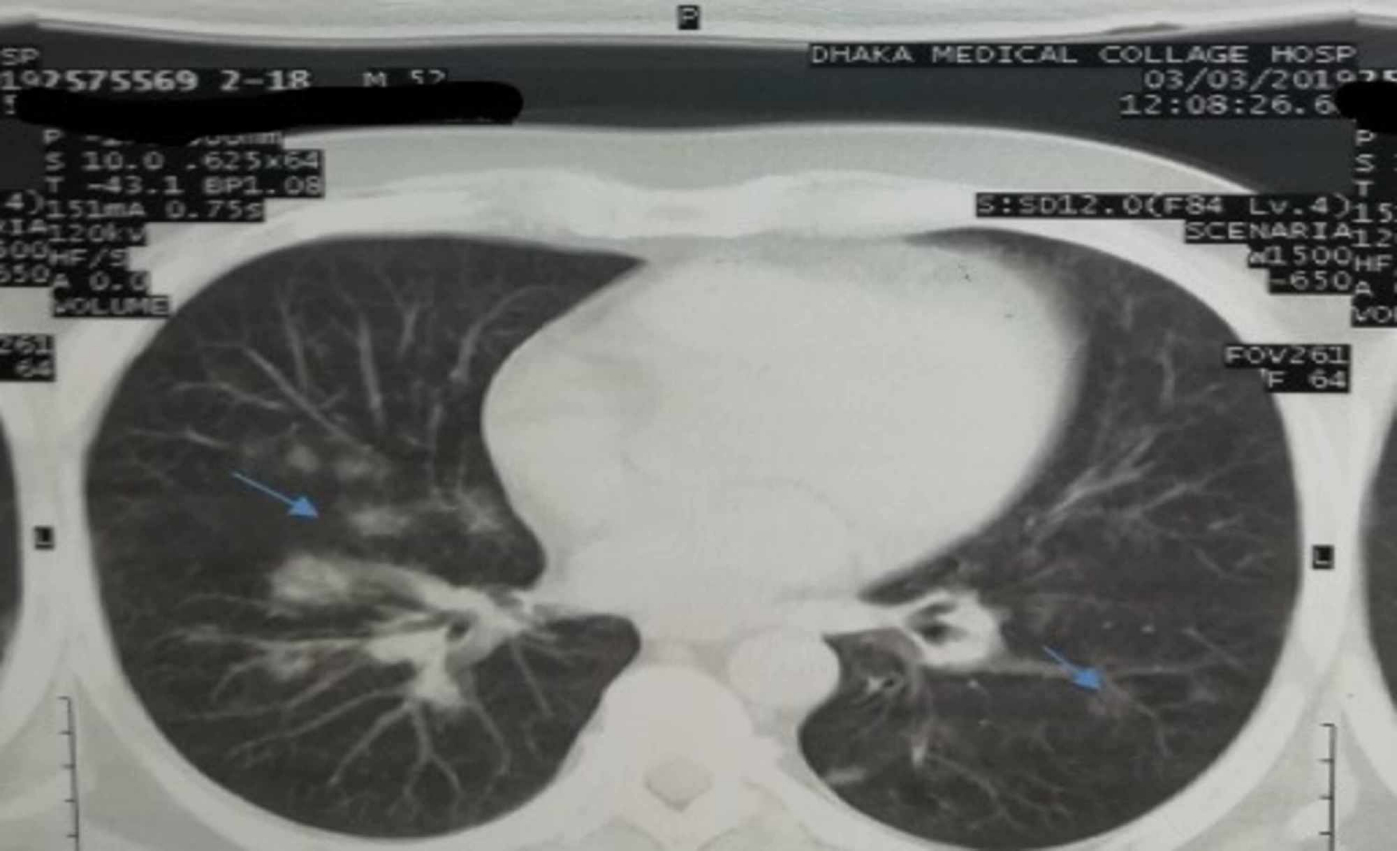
CT scan of the chest showing multiple dense nodular shadows with some cavitation involving upper and middle lobes of both lungs. The blue arrows are indicating inhomogeneous patchy opacities and cavitation throughout the lung.
